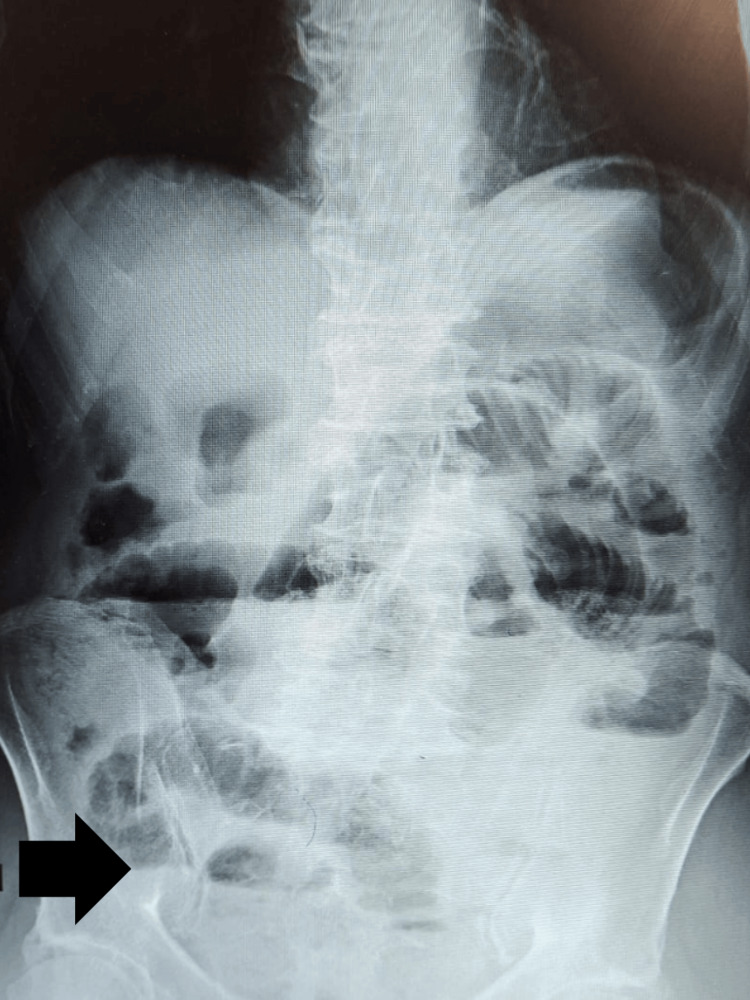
Erect abdominal radiograph demonstrating multiple air–fluid levels. The black arrow highlights a representative air–fluid interface.
